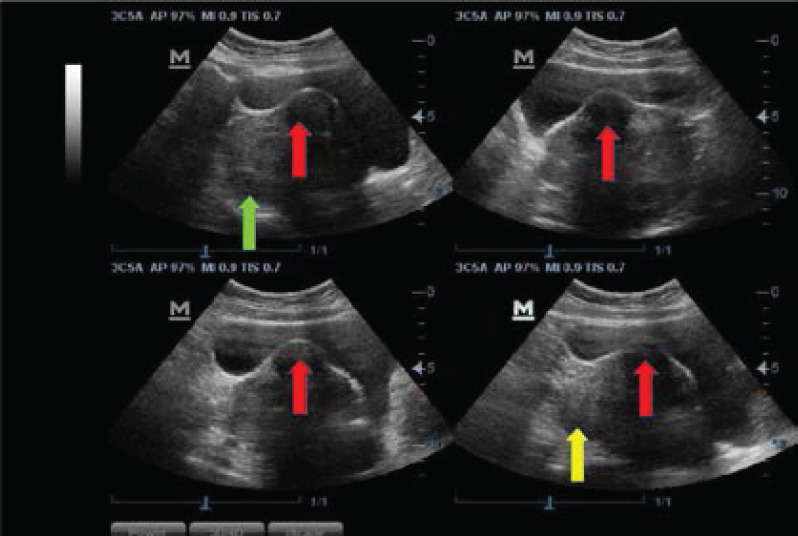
Sonograms showing anterior intramural uterine body myoma indenting into the posterior wall of the urinary bladder (red arrows), posterior uterine body myoma (green arrow), and a posterior uterine fundus (yellow arrow)
